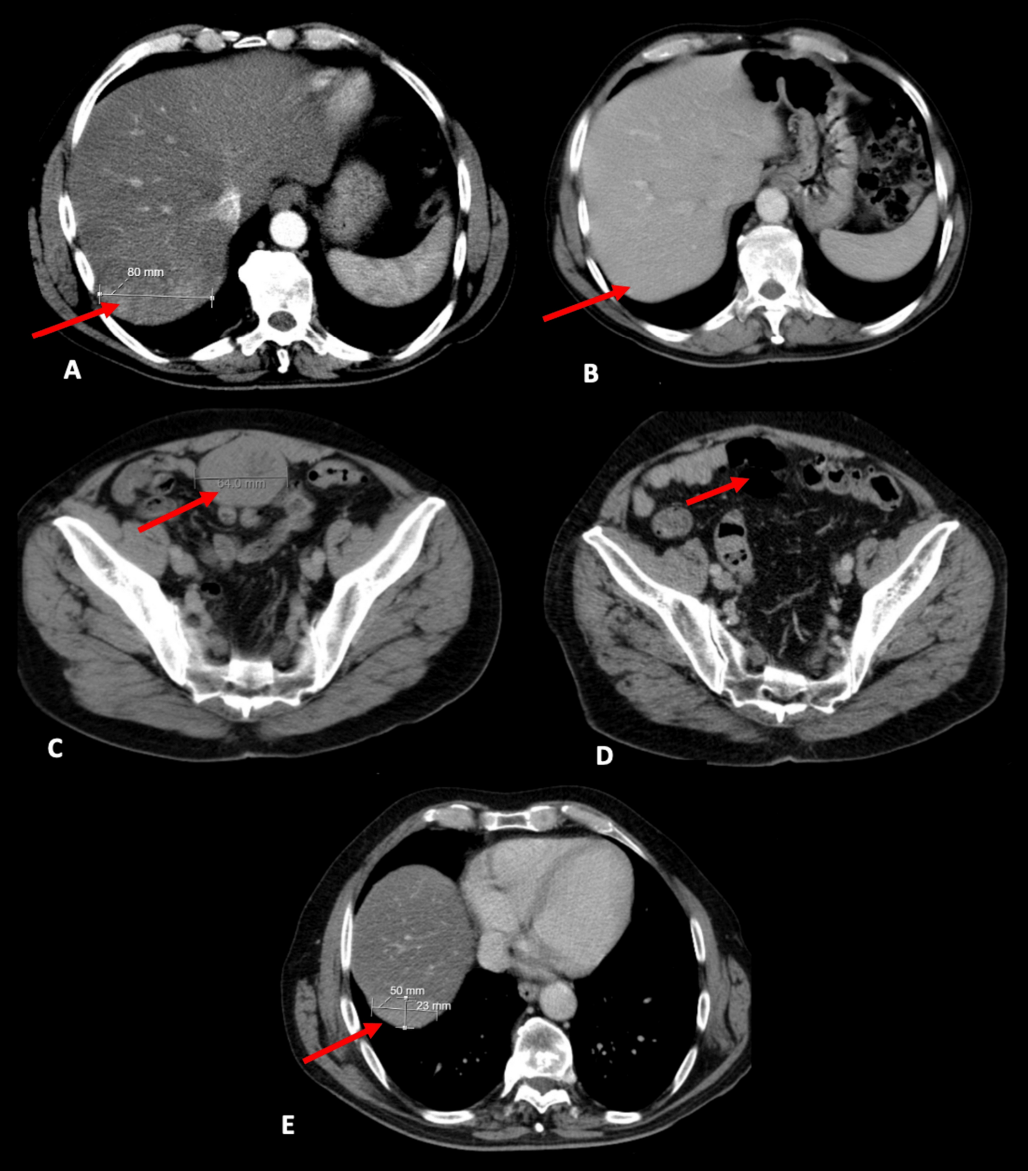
Computed tomography images of the gastrointestinal stromal tumor. (A) In 2007, prior to imatinib administration, a lesion emerged measuring 80 mm in the posterior right lobe of the liver. (B) By 2009, routine CT scans indicated the liver lesion had vanished. (C) In 2011, a novel pelvic lesion was observed. (D) By 2018, after six years of imatinib treatment, the pelvic mass had resolved. (E) In 2019, the disease recurred manifesting as a new lesion positioned above the diaphragm in proximity to the liver.
